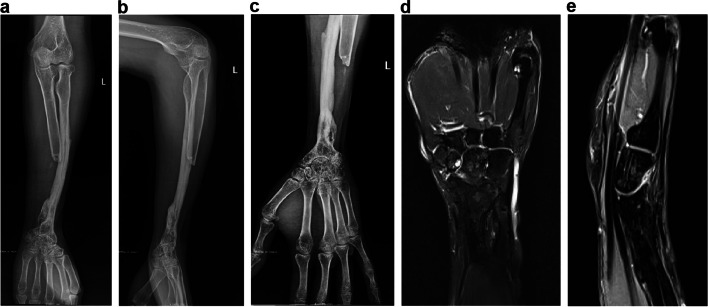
a-c Radiographs show a stable wrist and elbow and considerable hypertrophy of the fibula graft. d, e No signs of tumor recurrence are seen on magnetic resonance imaging
